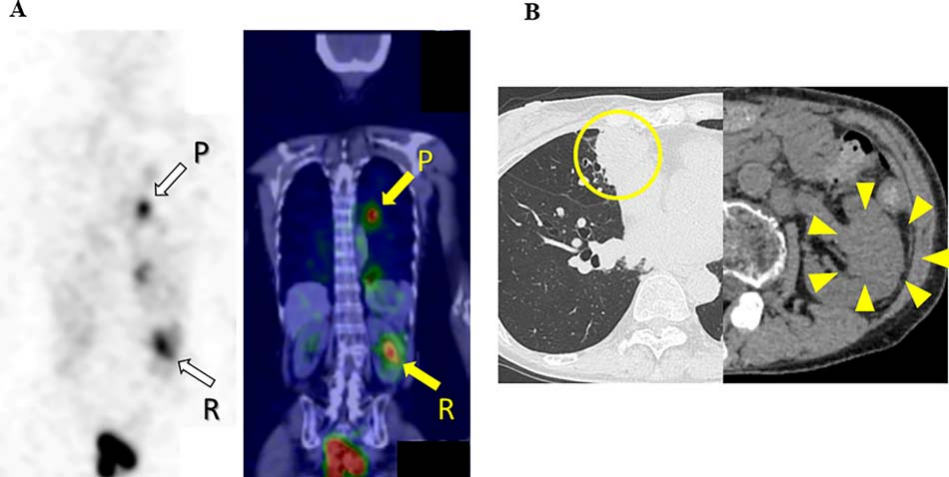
131I scintigraphy and CT images of the chest and abdomen after the second surgery; (A) in 131I scintigraphy, some lung metastases showed radioiodine uptake, whereas others did not. Radioiodine uptake was also observed in the left renal tumor, but not in the right kidney; left: RAI scintigraphy, right: SPECT–CT, P: pulmonary metastases, R: renal metastasis; (B) pulmonary and renal metastases both increased in size.
